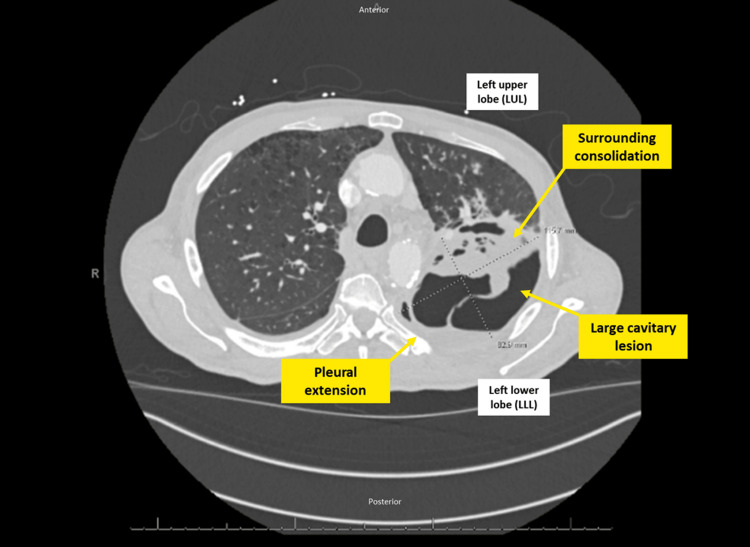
Computed Tomography (CT) of the chest revealing a large, complex necrotic cavity The lesion involves the apicoposterior segment of the left upper lobe and extends into the superior segment of the left lower lobe. There is evidence of direct erosion into the pleural space.
